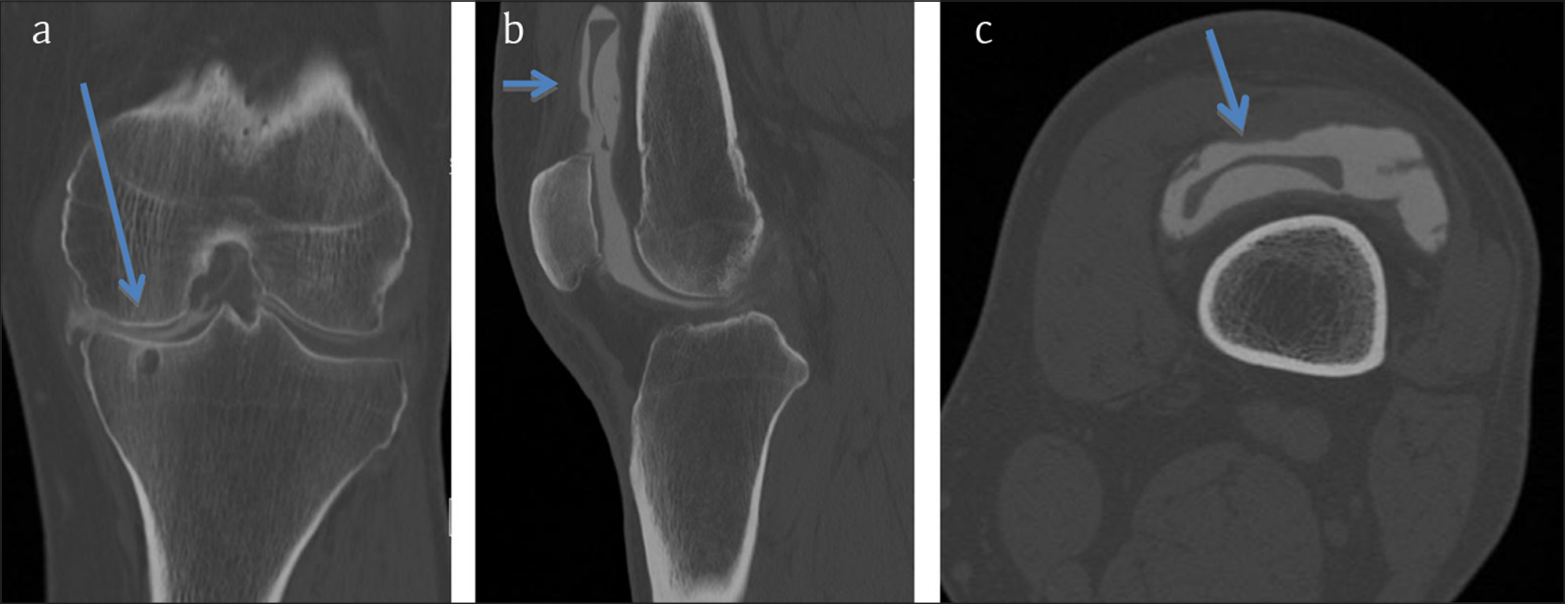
Arthro-CT with coronal (a), sagittal (b) and axial (c) views.
